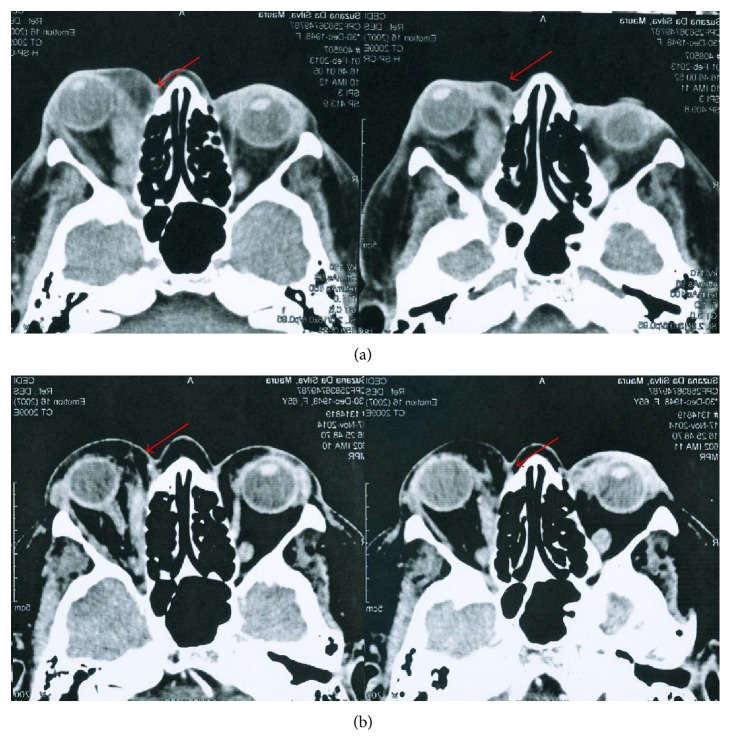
CT scan showing diffuse infiltrate of orbital tissue and muscle inflammation. Compare stages before (a) and after (b) immunosuppressive treatment.
